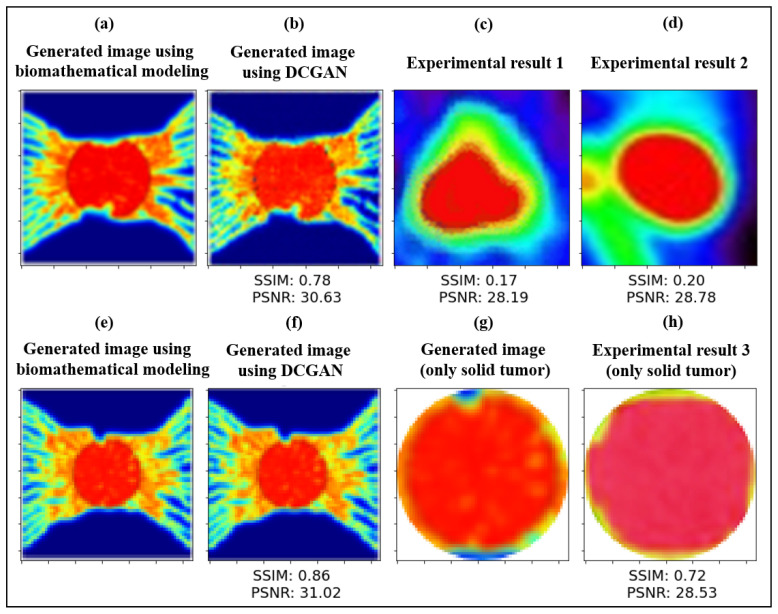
Quantitative and qualitative comparisons of the synthetic and clinical 18F-FDG PET radiotracer images of solid tumors at different stages of progression and angiogenesis. (a) A synthetic PET image generated by biomathematical modeling for a tumor with 2.4 cm diameter is compared with (b) an augmented PET image generated using the DCGAN model, and (c,d) two clinical PET images [58,57]. Comparison between (e) a synthetic PET image generated by biomathematical modeling without implementing the DCGAN for a 2cm tumor and (f) another augmented PET image generated using DCGAN. (g) The solid tumor in panel (f) is also compared with (h) another image of solid tumor taken experimentally [59], showing excellent agreement in terms of quantitative assessments with SSIM and PSNR values and qualitative comparison of radiotracer uptake in solid tumor. SSIM and PSNR values are defined according to Equations (16) and (17), respectively. Images in panels (c,d,h) are adopted and printed with permission from [57,58,59]. Copyright 2022.
